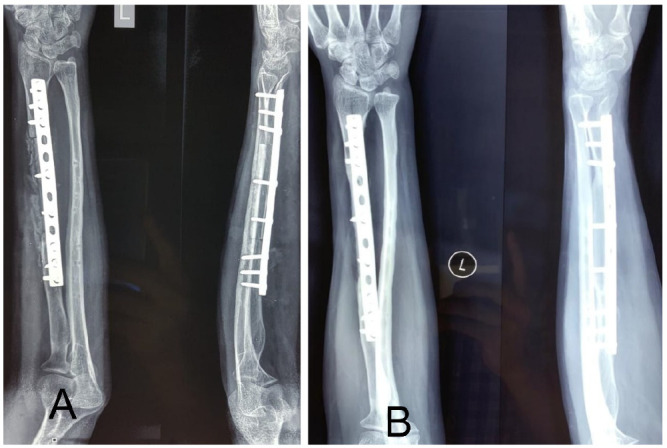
Post-operative radiographs: (A) Immediate Post op (B) 6 months follow up
